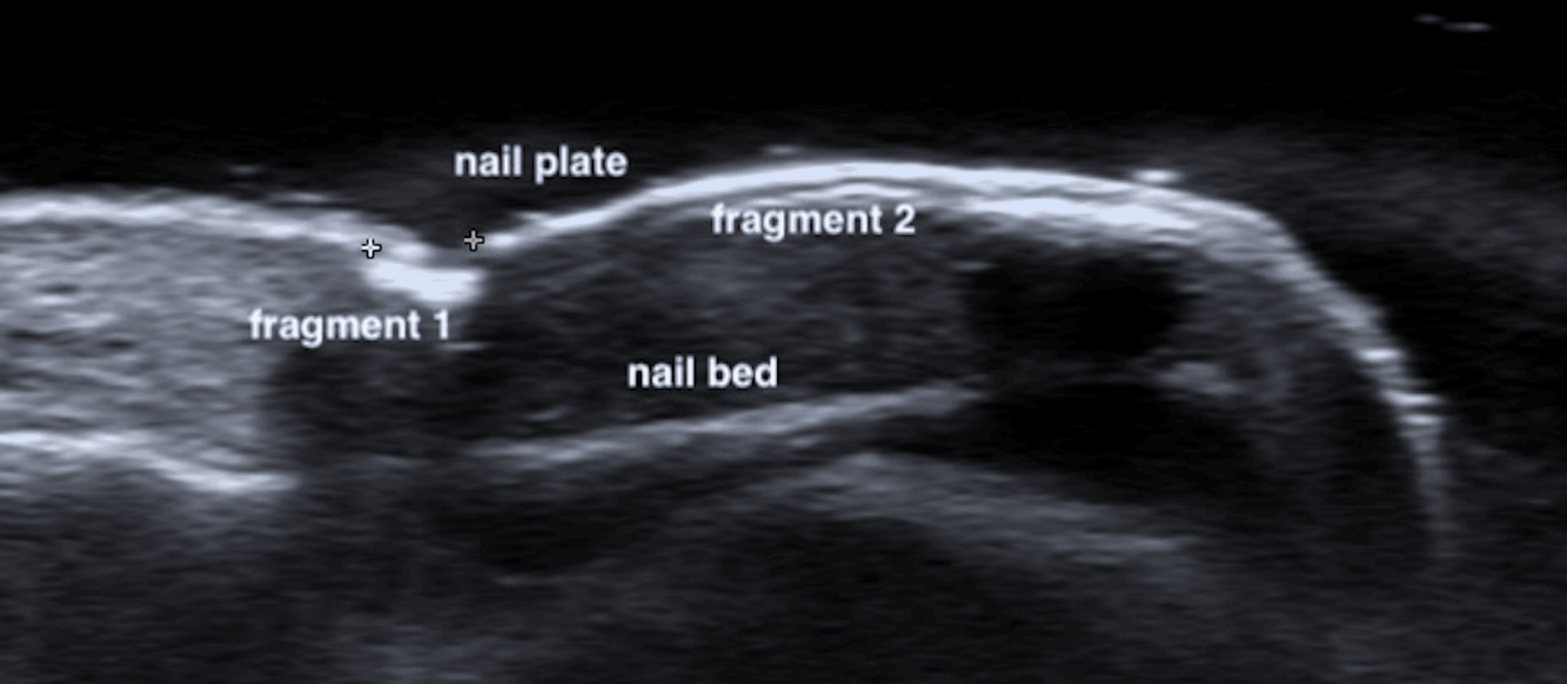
Ultrasound features of onychomadesis Longitudinal greyscale image showing multifragmentary onychomadesis, with two distinct fragments of the nail plate. The first fragment of onychomadesis is delimitated by the + signs. Original unpublished image, courtesy of Dr. Claudia Gonzalez.
